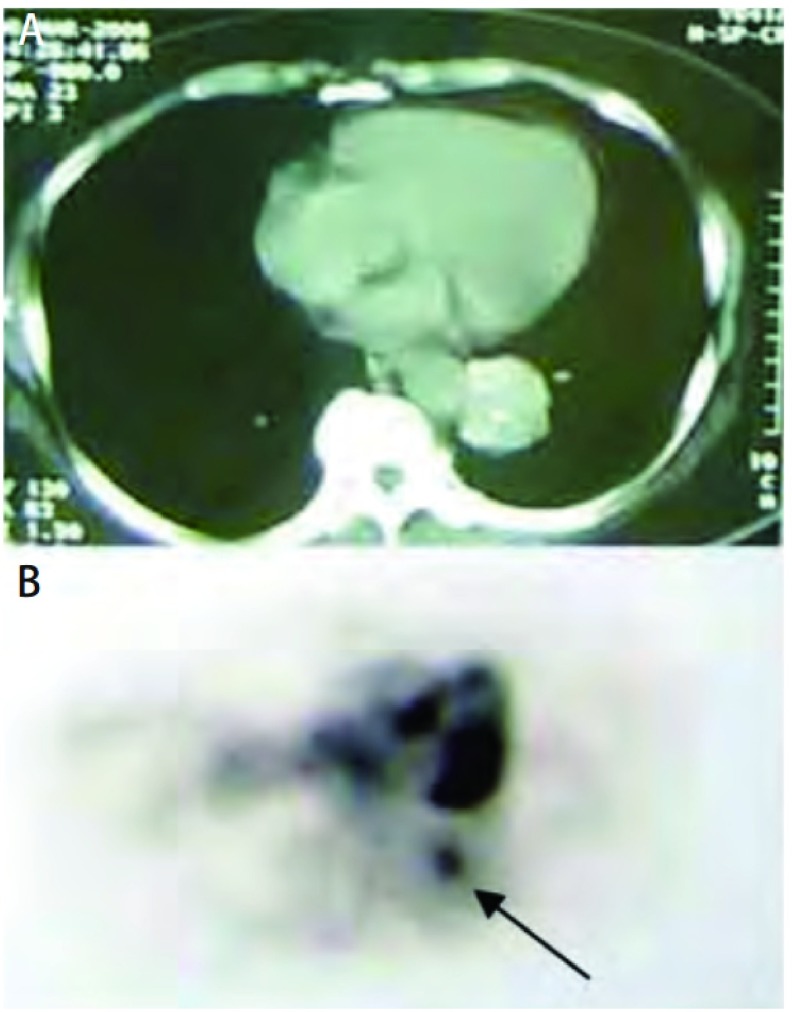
患者女性，74岁，慢性咳嗽。A：CT示左肺下叶35 mm×30 mm肿物，边界欠清，其内可见钙化；B：PET示高代谢灶(如箭头所示)。 Female patient, 74 years old, with chronic cough. A: CT shows left lower lobe 35 mm×30 mm mass, boundary is less clear and calcification; B: PET shows hypermetabolic lesion (arrow).
